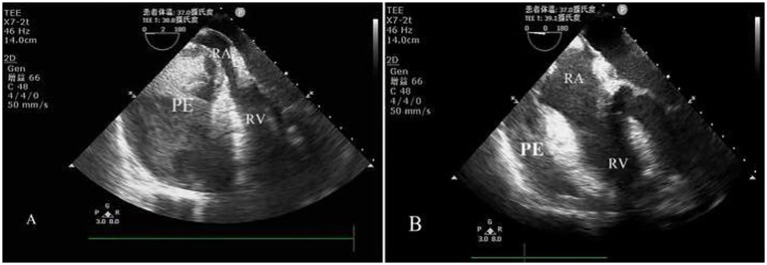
Four-chamber view on transesophageal echocardiography (TEE). (A) A large loculated pericardial effusion (PE) and the heart chambers on the right side collapsed, especially the right atrium (RA); RV, right ventricle. (B) Pericardial effusion (PE) decreased significantly, and the right atrium (RA) was refilled after subxiphoid surgical pericardial window and drainage; RV, right ventricle.
